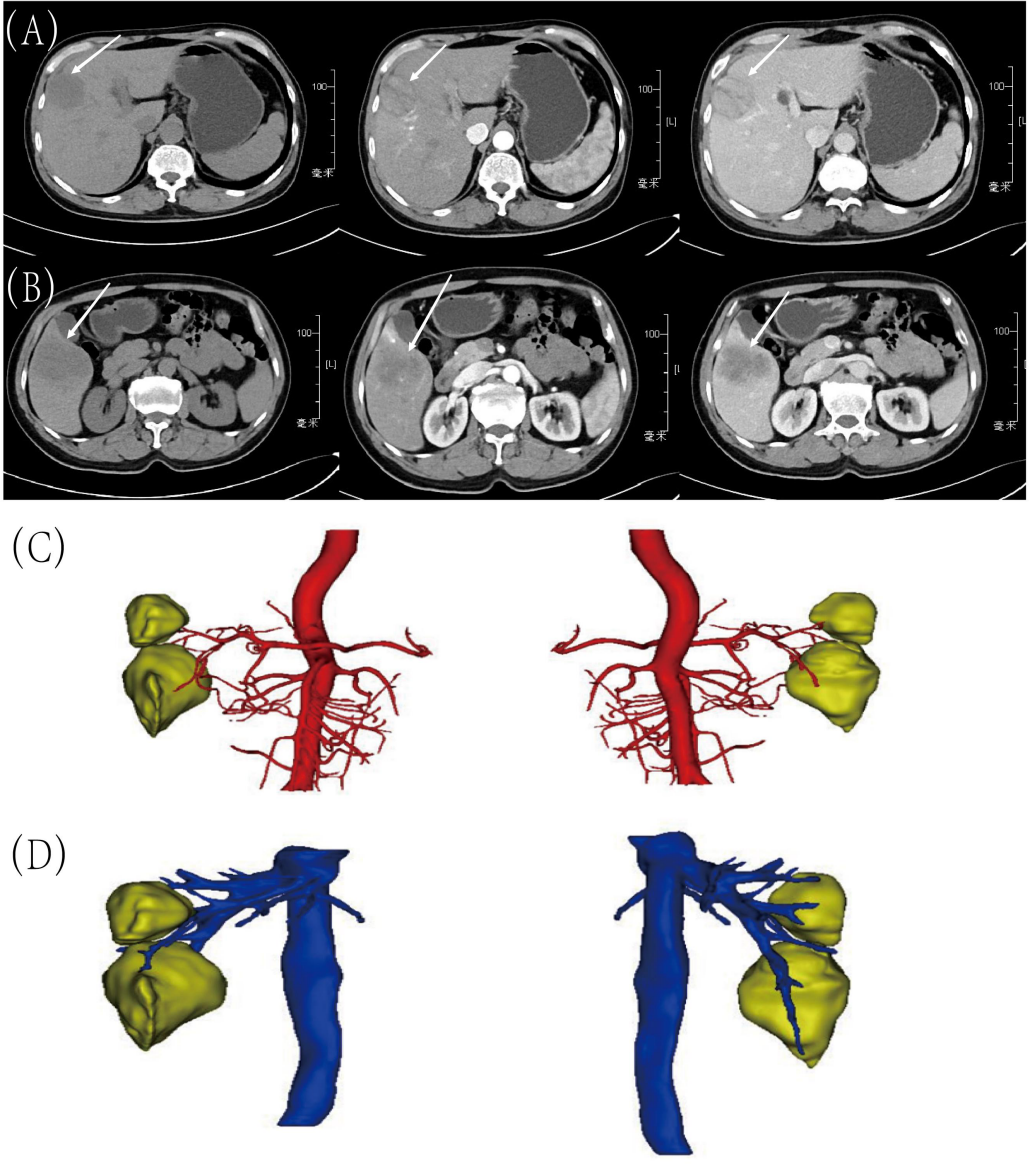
Preoperative CT images and Three-Dimensional Reconstructed CT. (A) Tumor in S8: before contrast, arterial phase and portal venous phase; (B) Tumor in S5: before contrast, arterial phase and portal venous phase; (C) Arterial relationships with tumors on 3D Reconstructed Images; (D) The relationship between veins and tumors on 3D Reconstructed Images.
